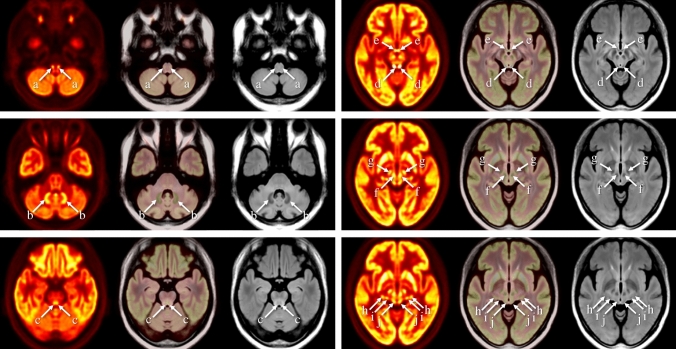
Average ^18^F-FDG PET (OSEM iteration 64 + TOF + PSF, left in each row), ^18^F-FDG PET/3D-FLAIR fusion (middle in each row), and 3D-FLAIR images (right in each row) (across hemispheres, *n* = 20). The average ^18^F-FDG PET image with OSEM iteration 64 + TOF + PSF reconstruction clearly shows FDG uptake in the olivary nuclei (a), dentate nuclei (b), midbrain raphe nuclei (c), inferior colliculi (d), mammillary bodies (e), red nuclei (f), subthalamic nuclei (g), lateral geniculate nuclei (h), medial geniculate nuclei (i), and superior colliculi (j)
